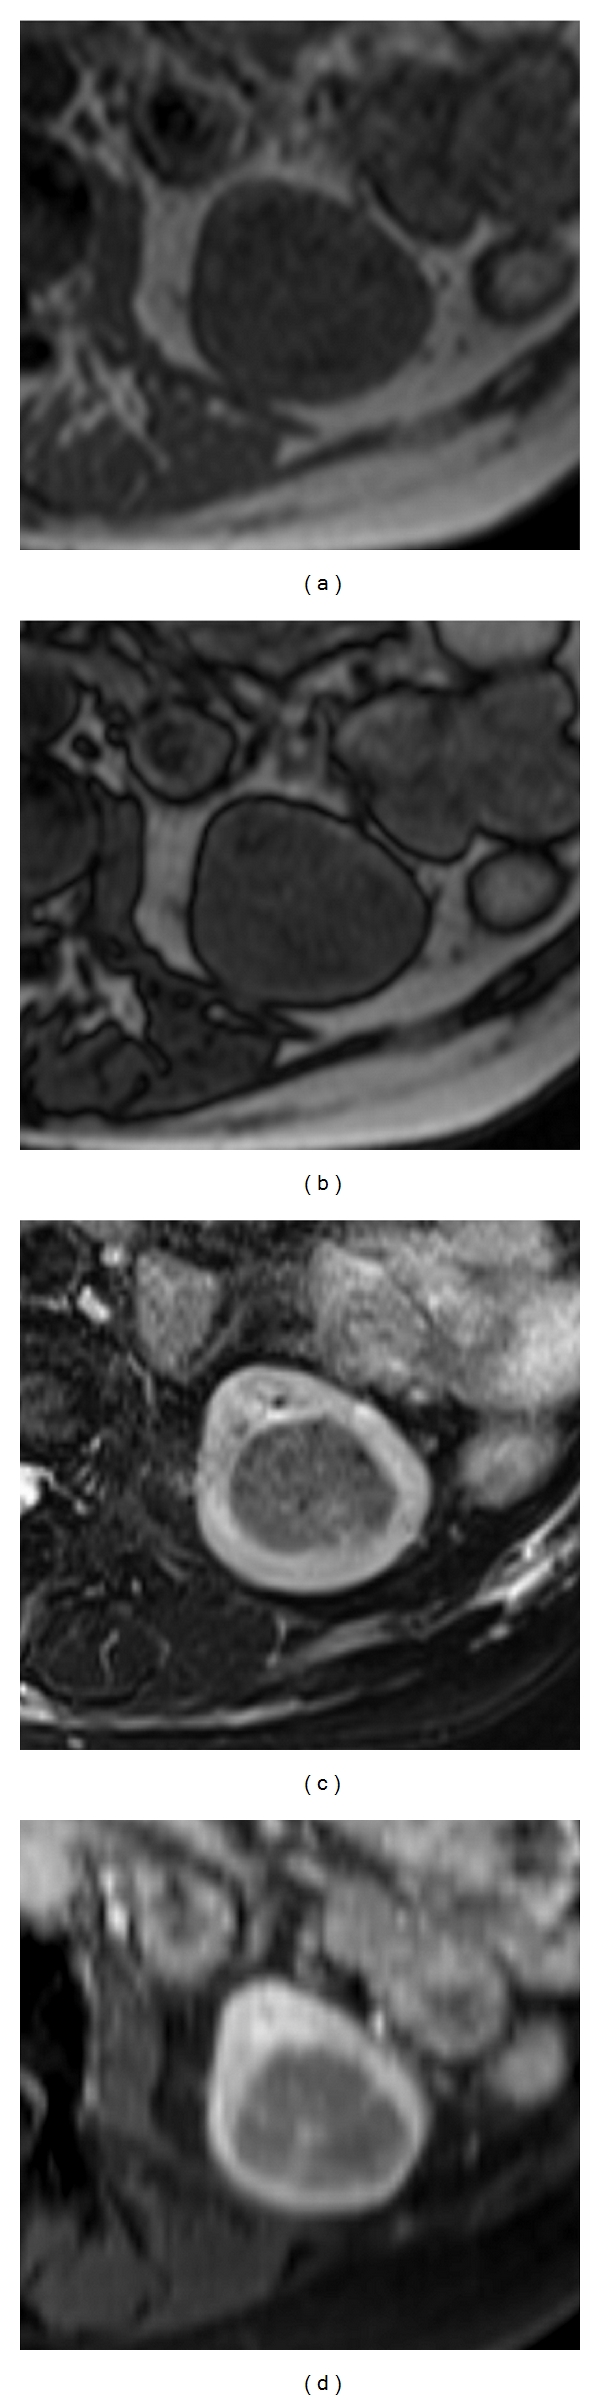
(a–d) Axial MR images of the left kidney, T1-weighted in-phase (a) T1-weighted opposed phase (b) T2-weighted fat-saturated (c), and T1-weighted, contrast-enhanced fat-saturated (d). The left renal mass is isointense in T1-weighted imaging without evidence of fatty components (a, b); the small hypointense spots in fat-saturated T2-weighted imaging are vessels (c). The mass is hypointense in T1-weighted imaging after i.v. contrast media (d); solley centrally there are KM-enhancing foci representing vessels (d).
